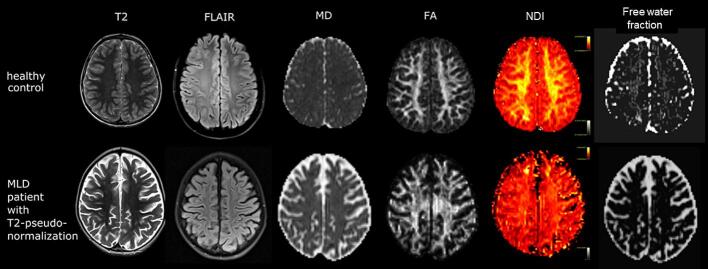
Healthy control (upper row) and a patient with metachromatic leukodystrophy (MLD, lower row) with normal T2-weighted signal intensity in cerebral white matter, which was T2-hyperintense in previously obtained MR scans. Besides DTI-parameters such as MD and FA, NODDI can model the neurite density index (NDI) and the free water fraction. The neurite density is clearly reduced in the normal looking white matter in the MLD patient, confirming pseudo-normalization.
